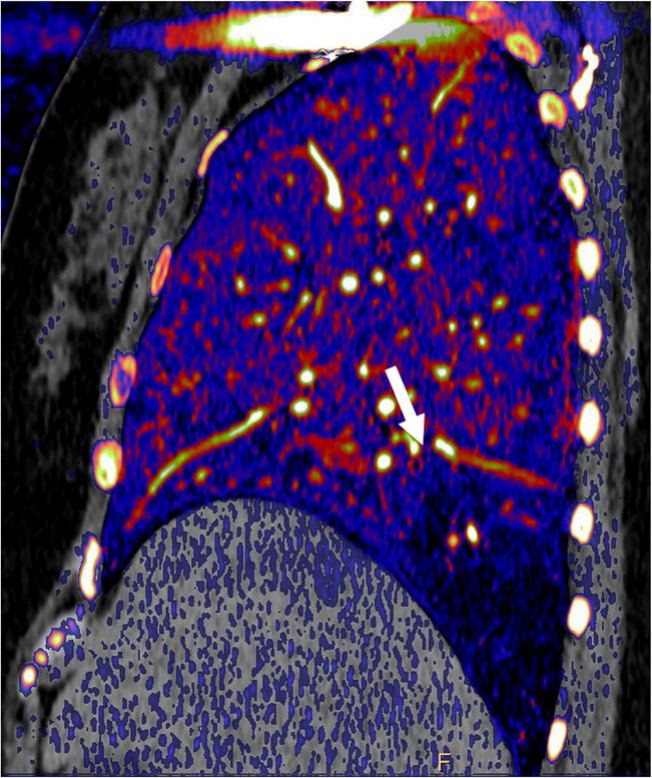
Perfusion imaging with iodine maps. Sagittal iodine overlay image in a patient with acute chest pain shows a wedge-shaped perfusion defect in the right lower lobe (*arrow*), which is consistent with an acute pulmonary embolism. CTA in this patient (not shown here) did not show the clot clearly
